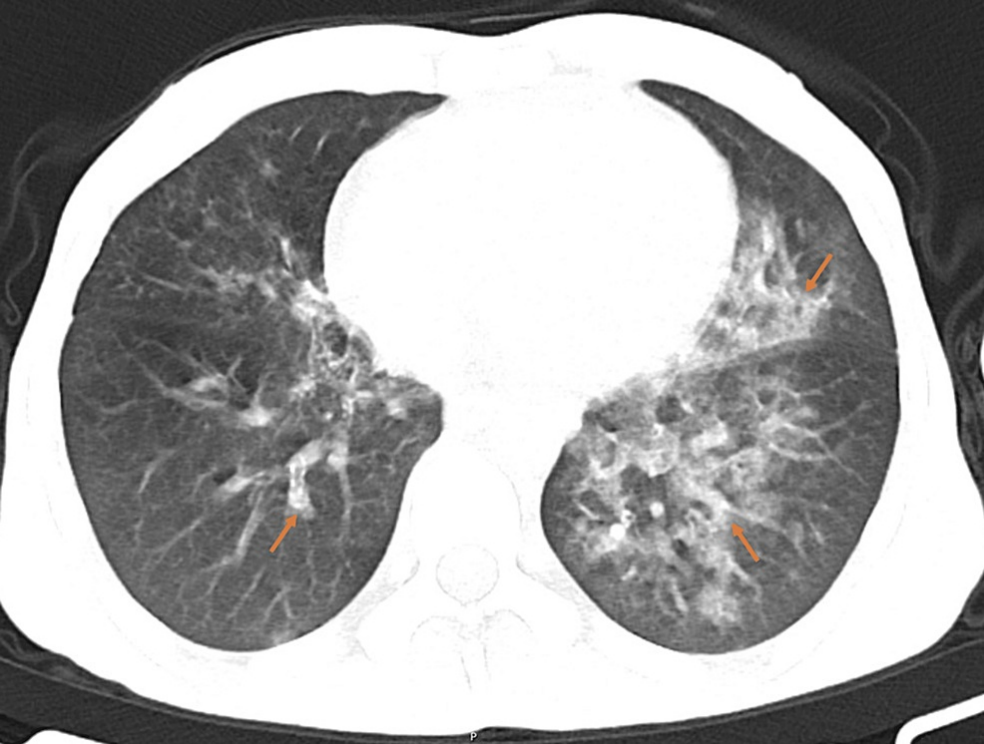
Axial computed tomography (CT) scan of the thorax showing bilateral patchy ground-glass opacities (arrows).
